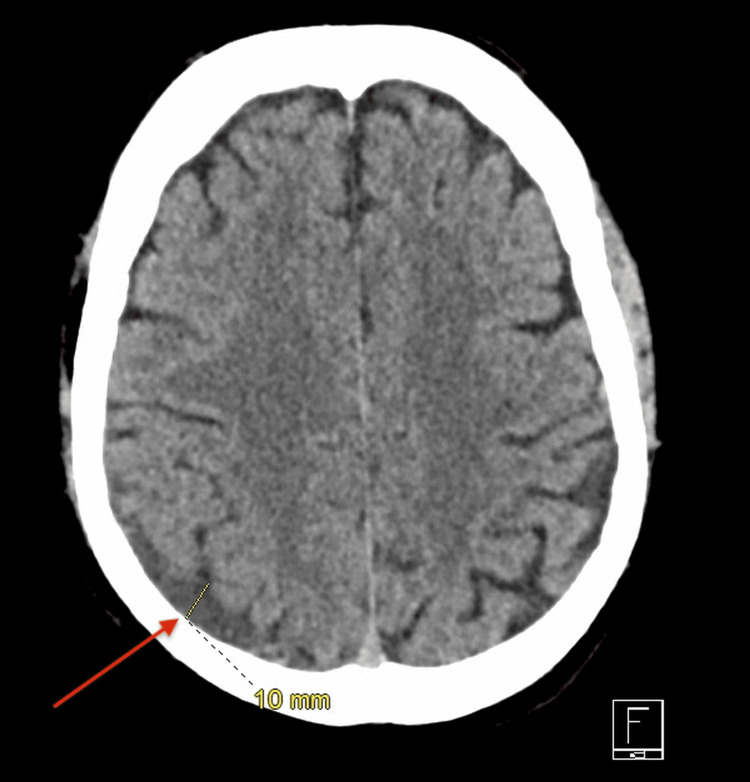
Noncontrast CT of the brain demonstrating a chronic subdural hematoma Axial CT demonstrating a small chronic right posterior parietal subdural hematoma (red arrow) measuring approximately 10 mm in maximal thickness, with internal punctate hyperdensities representing calcification versus a small acute-on-chronic hemorrhagic component. This finding was radiographically distinct from the pineal-region lesion and was not considered the primary driver of the patient’s endocrine presentation.
